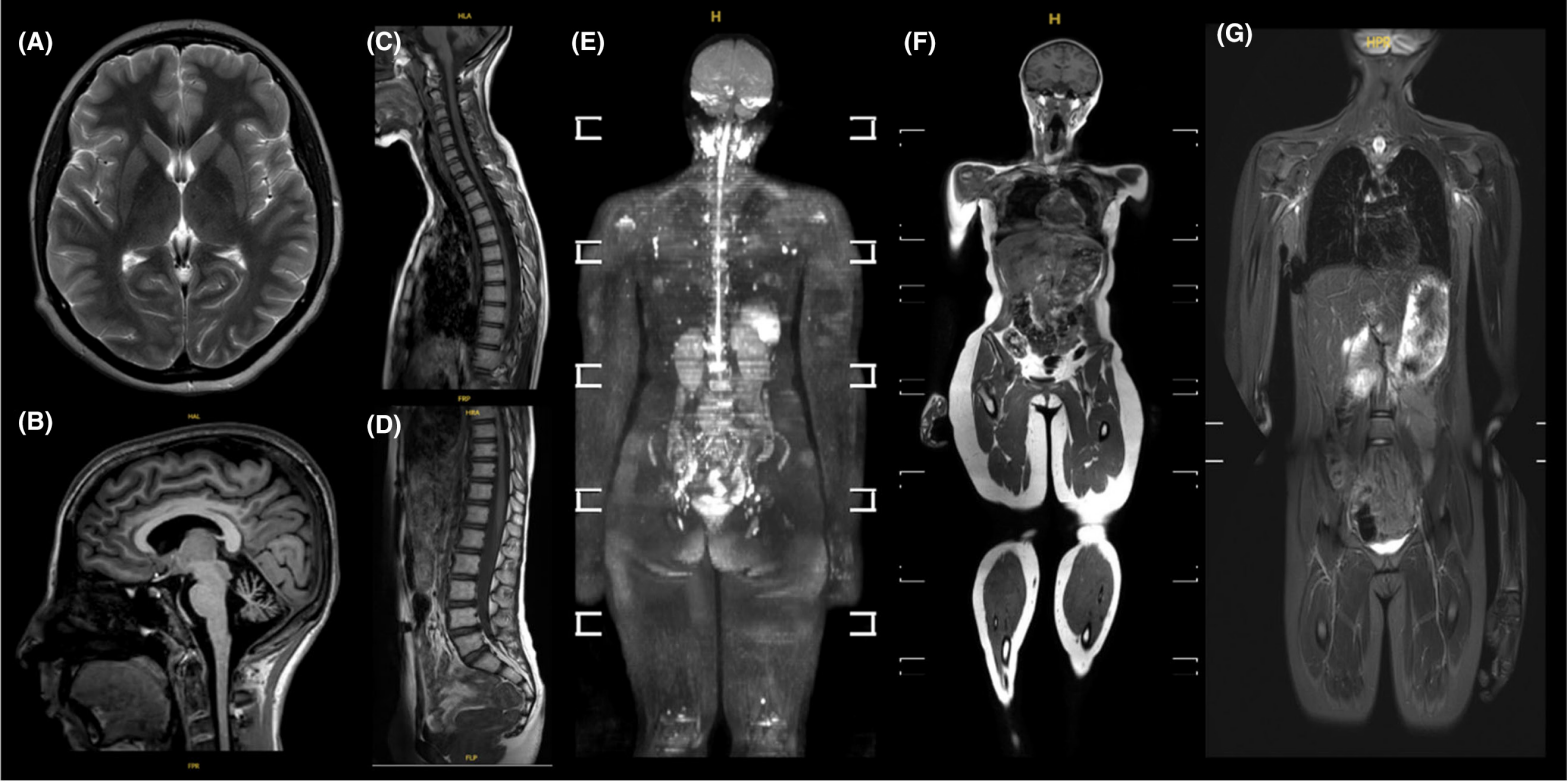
Examples of MRI scans with image quality classified between very good to excellent: (A) axial T2‐weighted brain image; (B) 3D T_1_‐weighted brain image; (C) and (D) sagittal T_1_‐weighted whole‐spine; (E) whole‐body 3D‐rotational MIP b900 DWI; (F) whole‐body coronal T_1_‐weighted mDIXON and (G) whole body coronal STIR.
